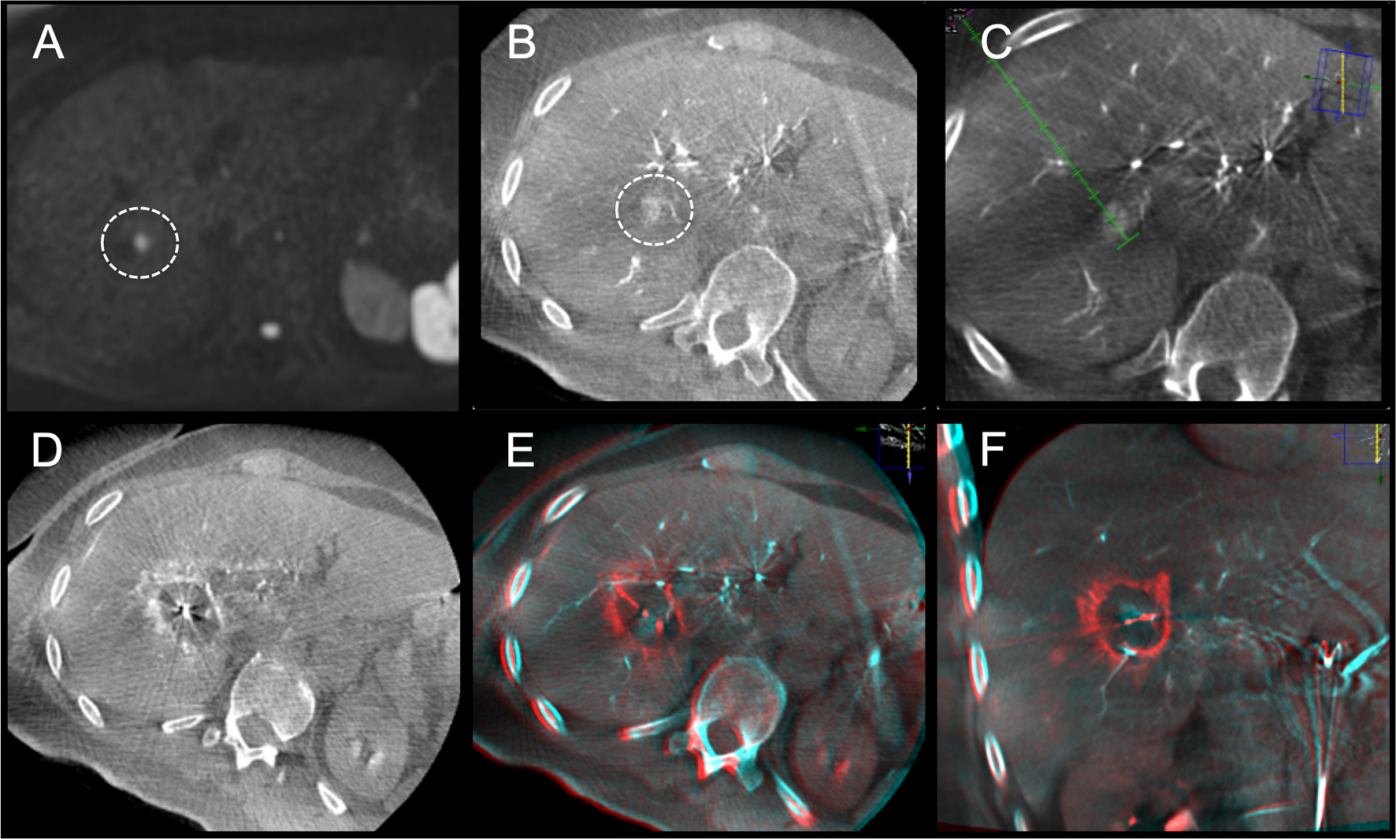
Example of a colorectal liver metastasis ablation. A. Solitary colorectal cancer liver metastasis only visible on diffusion weighted MRI (not visible on contrast-enhanced MRI). B. C-arm CT with hepatic arteriography clearly depicts the 11 mm lesion. C. Planning of trajectory. D. Ablation zone depicted with C-arm CT hepatic arteriography directly after ablation for 8 min at 120W. E and F. Fused pre- and post-ablation C-arm CTs in axial and coronal view show adequate margins
